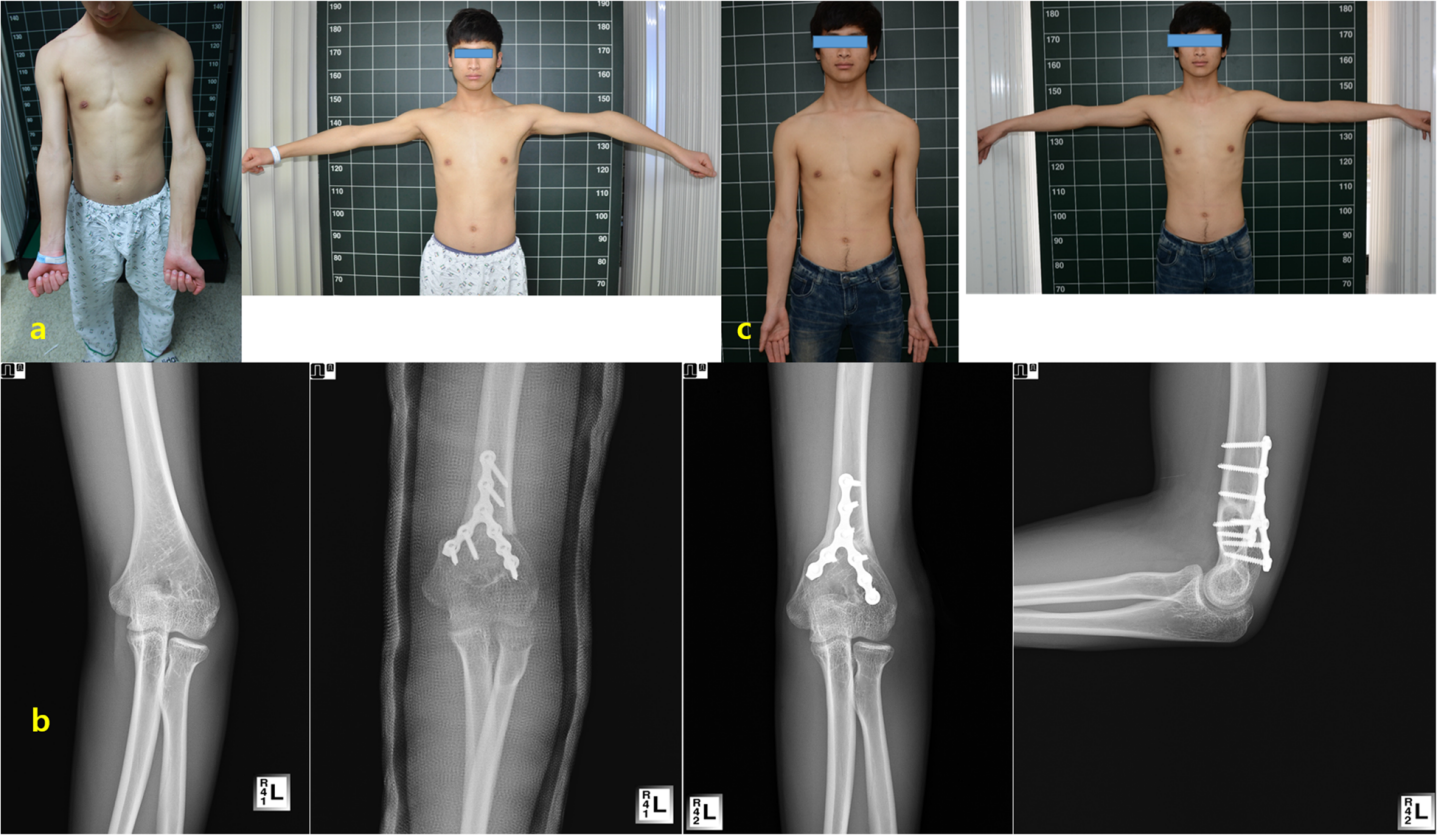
a A19-year-old male (Patient 17) who presented with a cubitus varus deformity; b Preoperative, postoperative, and last follow radiographs showing translation step-cut osteotomy of deformity with Y plate and screws; c At the last follow up, HEW angle and LPI were corrected to valgus 20° and 5%, respectively. He showed excellent results with an angle of elbow motion of 130° (extension, 0°; further flexion, 130°)
